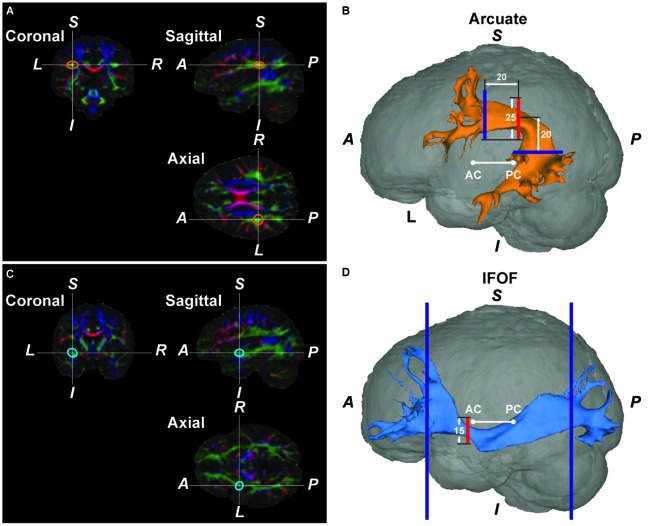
The Arcuate and inferior fronto-occipital fasciculus (IFOF) reconstructed by diffusion magnetic resonance imaging (MRI) in a typical participant. (A) A color-coded map of fiber bundles in the native space of a participant. In the Montreal Neurological Institute (MNI) space, we placed a “bottleneck mask” (a sphere of 10-mm radius) at a region where a narrower portion of the pathway was expected to pass. The mask was then transformed back to the native space. The orange ellipses denote the approximate outline of the transformed bottleneck mask on the left Arcuate. (B) The reconstructed left Arcuate. On a coronal slice, we generated a seed mask (shown in red), whose center was placed at the center of the transformed bottleneck mask on the left Arcuate. The waypoint masks are shown in blue. (C) A color-coded map of fiber bundles for the same participant. The blue ellipses denote the outline of the transformed bottleneck mask on the left IFOF. (D) The reconstructed left IFOF. On a coronal slice, we generated a seed mask (shown in red), whose center was placed at the center of the transformed bottleneck mask on the left IFOF. *A*, anterior; *P*, posterior;* S*, superior; *I*, inferior; *L*, left; *R*, right; AC, anterior commissure; PC, posterior commissure.
